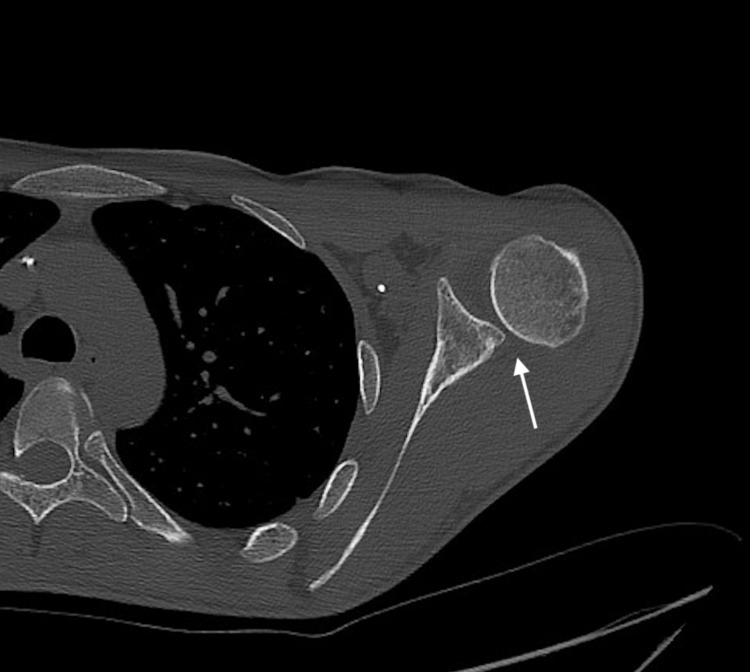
Axial CT shoulder showing posteroinferior subluxation CT: computed tomography
